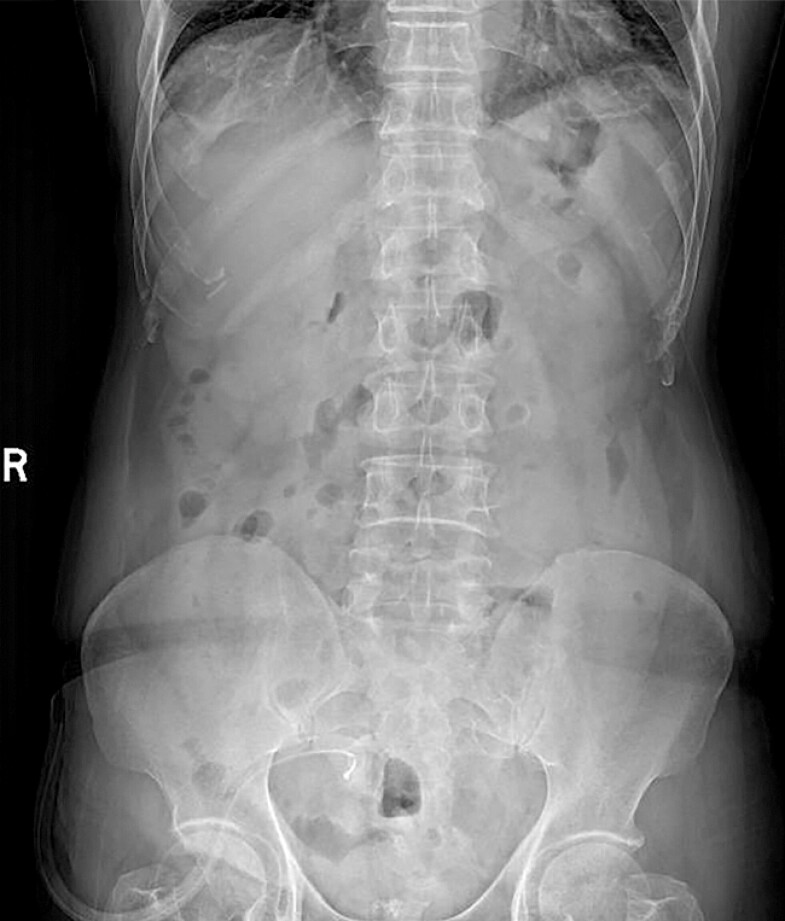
An unremarkable abdominal radiograph after stricturotomy.
